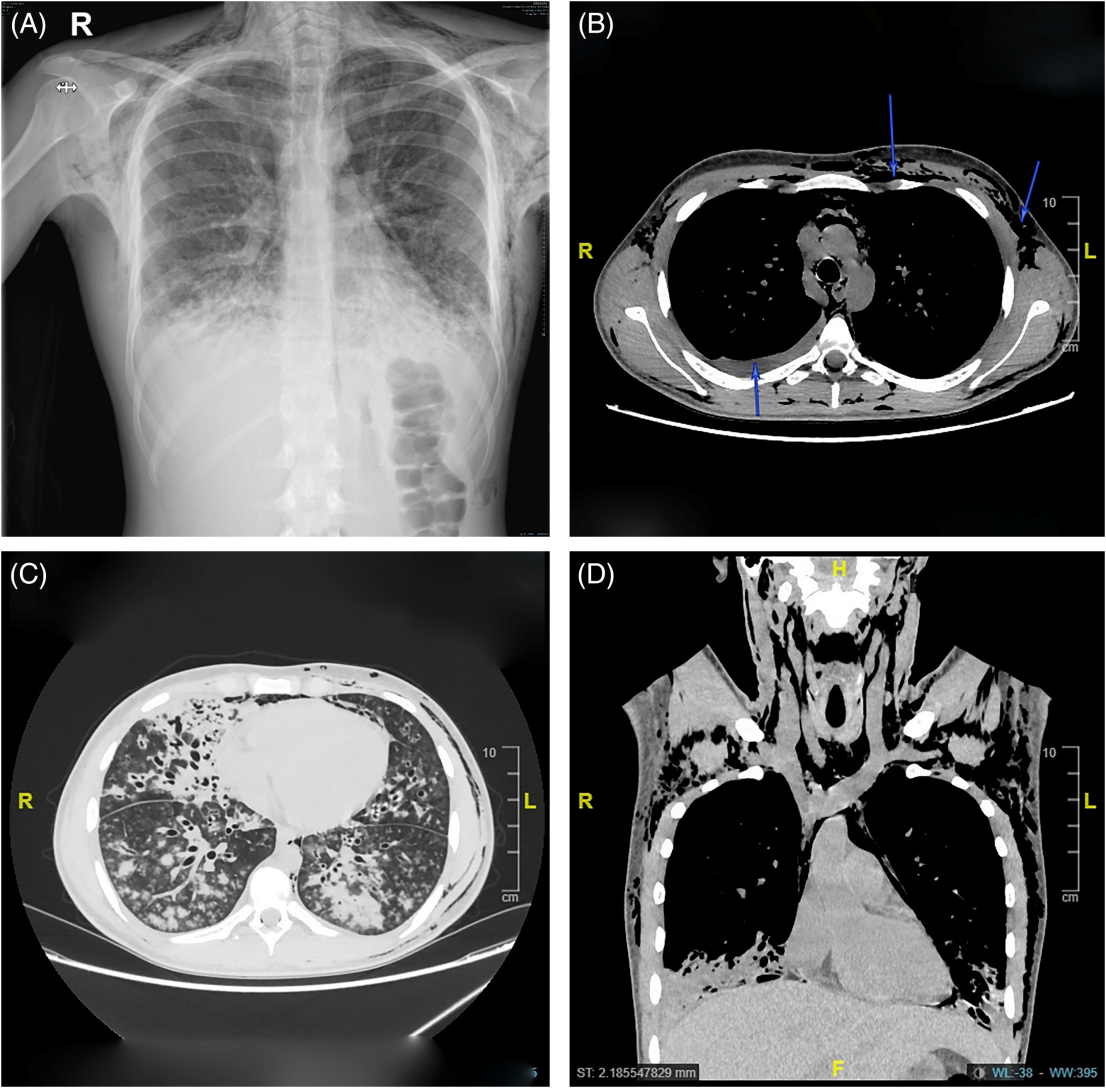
(A–D) presents the images captured on the first day. (A) The chest x‐ray revealed subcutaneous emphysema in the neck and chest. Furthermore, the pneumomediastinum and lung infiltrations bilaterally with obliteration of the costophrenic angles indicate pleural effusions. (B) Computed tomography chest shows subcutaneous emphysema, as indicated by the two arrows above, and pleural effusion, as indicated by the lower arrow. (C) Lung window shows a significant bilateral consolidation, indicating pneumonia and an infectious process. (D) It is a coronal section where we can see the subcutaneous emphysema in the chest, neck, and mediastinum. In addition, there is a consolidation in the lower lobe of the right lung.
